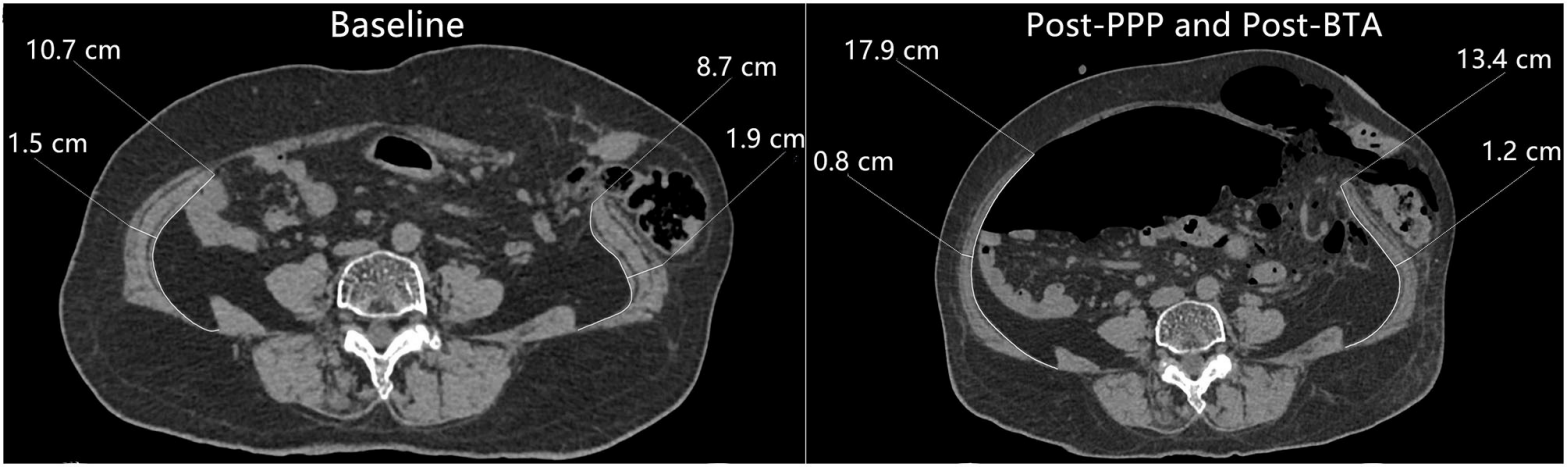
Comparison of preoperative axial computed tomography picture before and after preoperative techniques. There is significant elongation and thinning of the lateral abdominal muscles.
